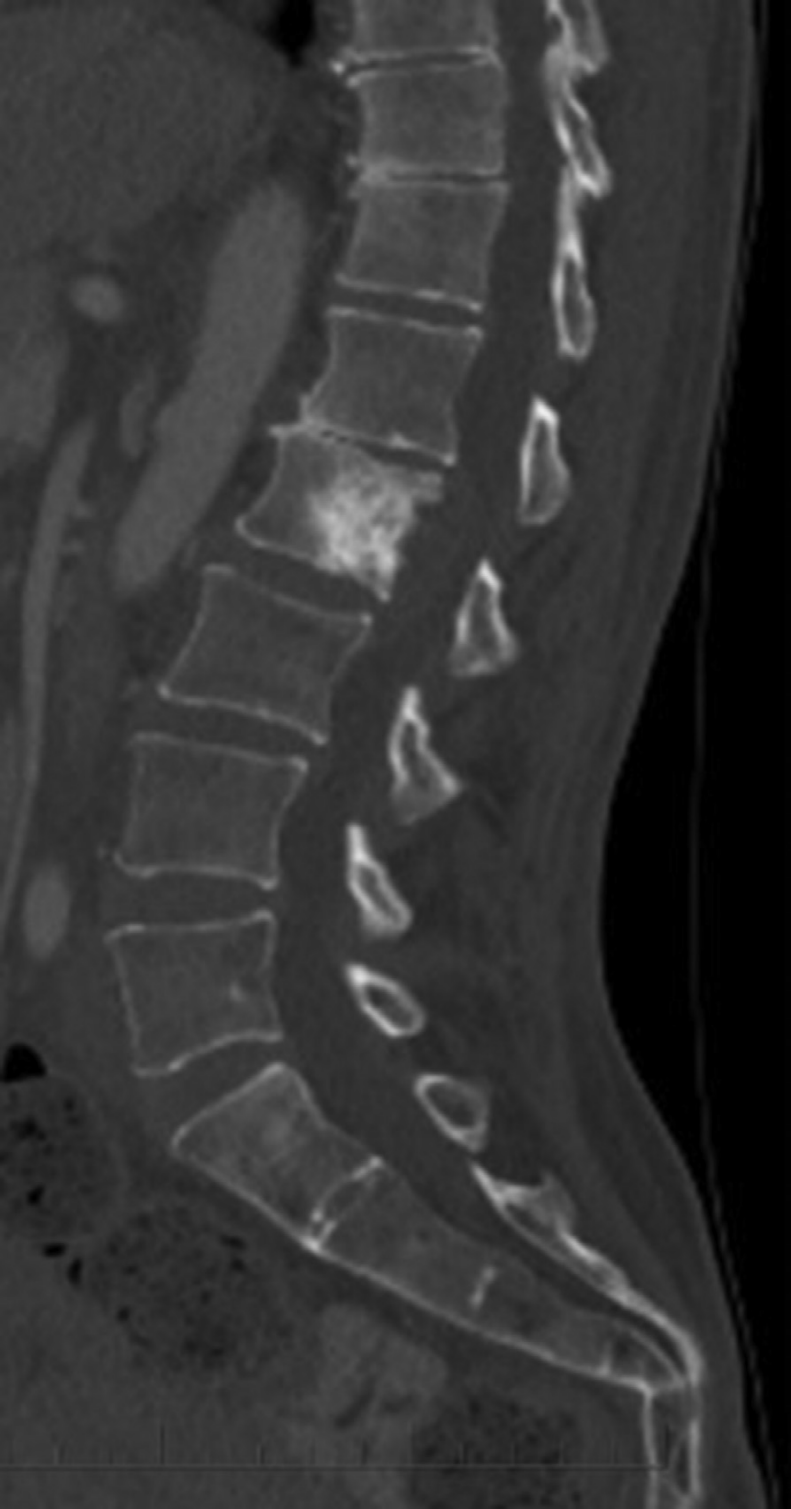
Mid sagittal CT image demonstrates a sclerotic lesion in the L2 vertebral body with irregular margins and destruction of the posterior cortex. No mineralization is seen in the epidural soft tissue component. No other suspicious lesion was identified on this CT of the chest, abdomen, and pelvis with IV contrast.
